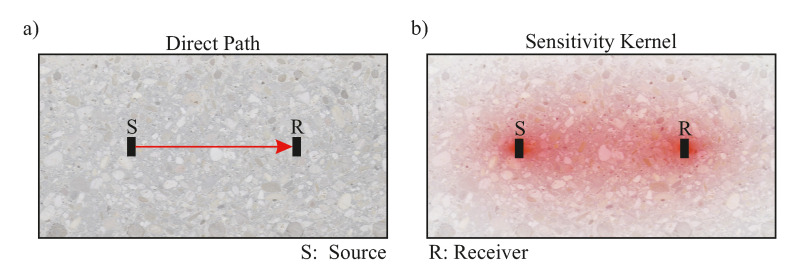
In classical ultrasonic (US) measurements, travel times are recorded and calculated via the ballistic waves. (a) Ballistic wave between source and receiver. Using coda waves, changes of travel time within a larger segment, described by (b) the sensitivity kernel (red), can be resolved.
